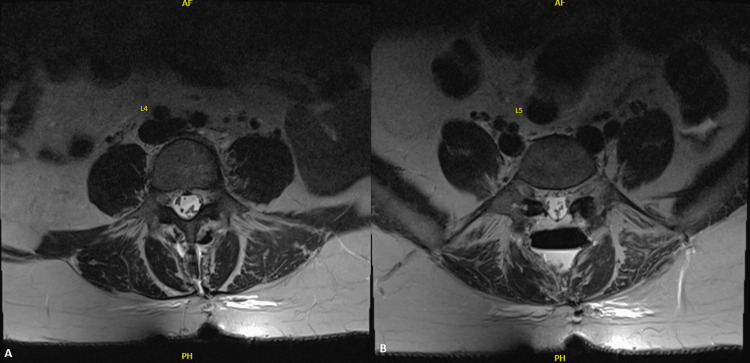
Postoperative T2 MRI transverse view. From left to right, these images demonstrate the changes seen after laminectomies and partial medial facetectomies at L4-L5. Image A is the 4th lumbar vertebra. Image B is the 5th lumbar vertebra. The nerve roots are now seen within the spinal canal without peripheral displacement.
